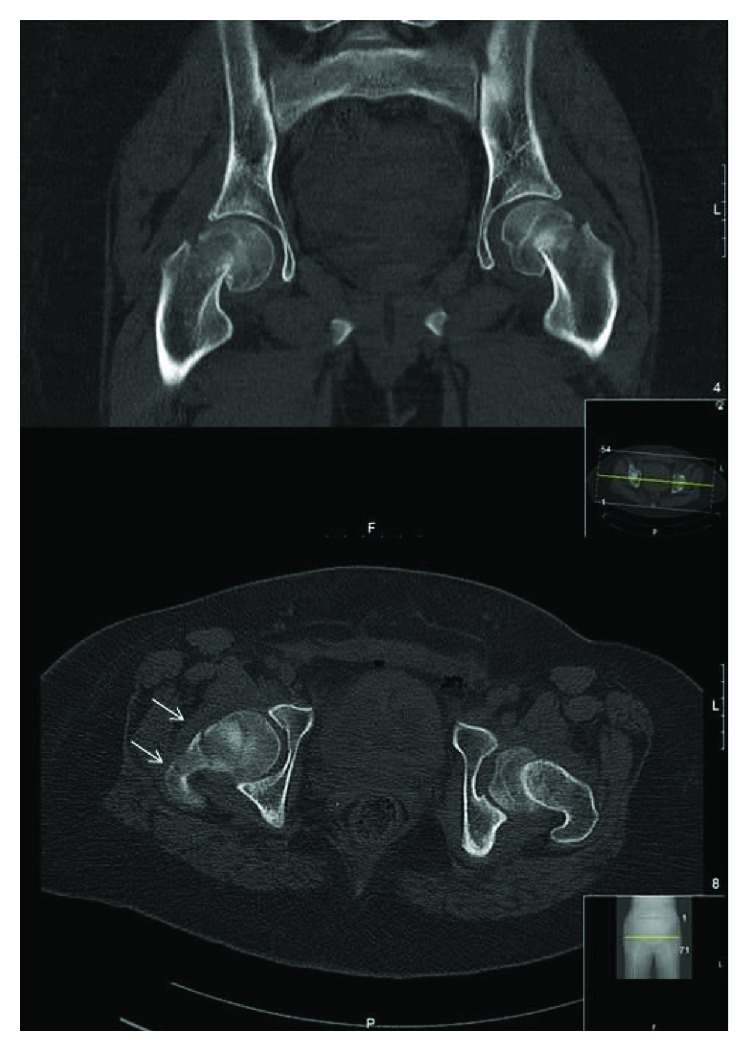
Computed tomography images (coronal and axial plane) confirmed not only the bilateral displacement of the femoral heads but also the presence of a 3-part fracture of the right side (arrows), which helped us to decide for bilateral total hip replacement than internal fixation.
